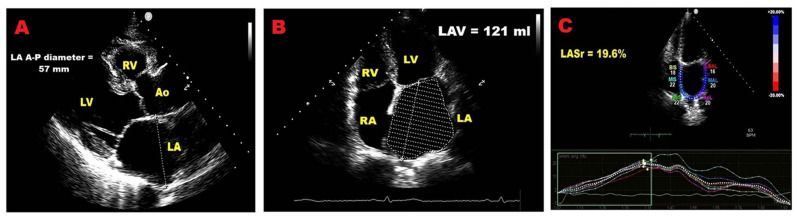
TTE and STE-derived LA indicators of adverse outcome in PMR individuals. (A) Enlarged LA diameter. (B) Increased LA volume. (C) Reduced LASr. A-P, antero-posterior; Ao, aorta; LA, left atrial; LASr, left atrial reservoir strain; LAV, left atrial volume; LV, left ventricular; PMR, primary mitral regurgitation; RA, right atrial; RV, right ventricular; STE, speckle tracking echocardiography; TTE, transthoracic echocardiography.
